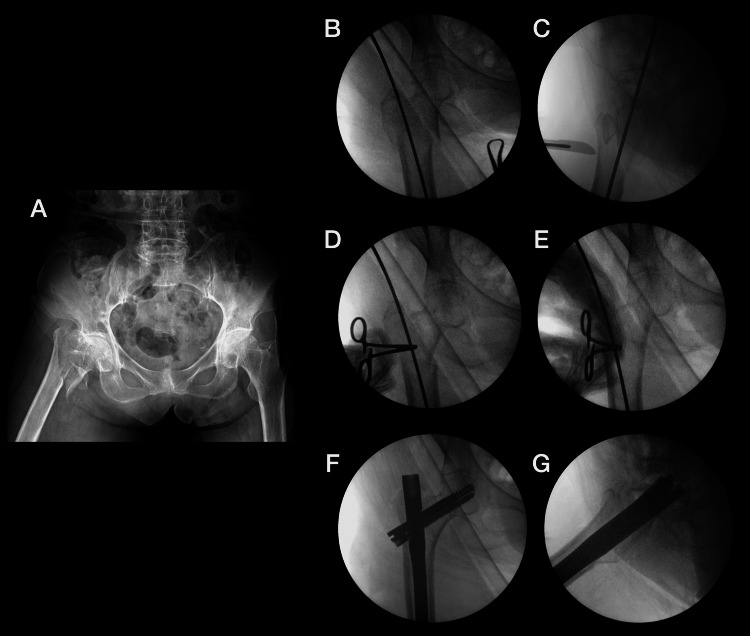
Sequential fluoroscopic assessment of intertrochanteric fracture fixation on a standard table (A) Preoperative anteroposterior radiograph of an 87-year-old female patient showing a right-sided unstable intertrochanteric femur fracture (AO/OTA 31-A3.3). (B, C) Intraoperative anteroposterior and lateral images confirm the identification of the entry point and preliminary alignment. (D, E) Closed reduction was performed using longitudinal traction and anterior percutaneous clamping, confirmed by fluoroscopy. (F, G) Final anteroposterior and lateral fluoroscopic images showing satisfactory reduction, restoration of a neck–shaft angle near 130°, and an acceptable tip–apex distance (15–25 mm) following cephalomedullary nail insertion. AO/OTA: AO Foundation/Orthopaedic Trauma Association
